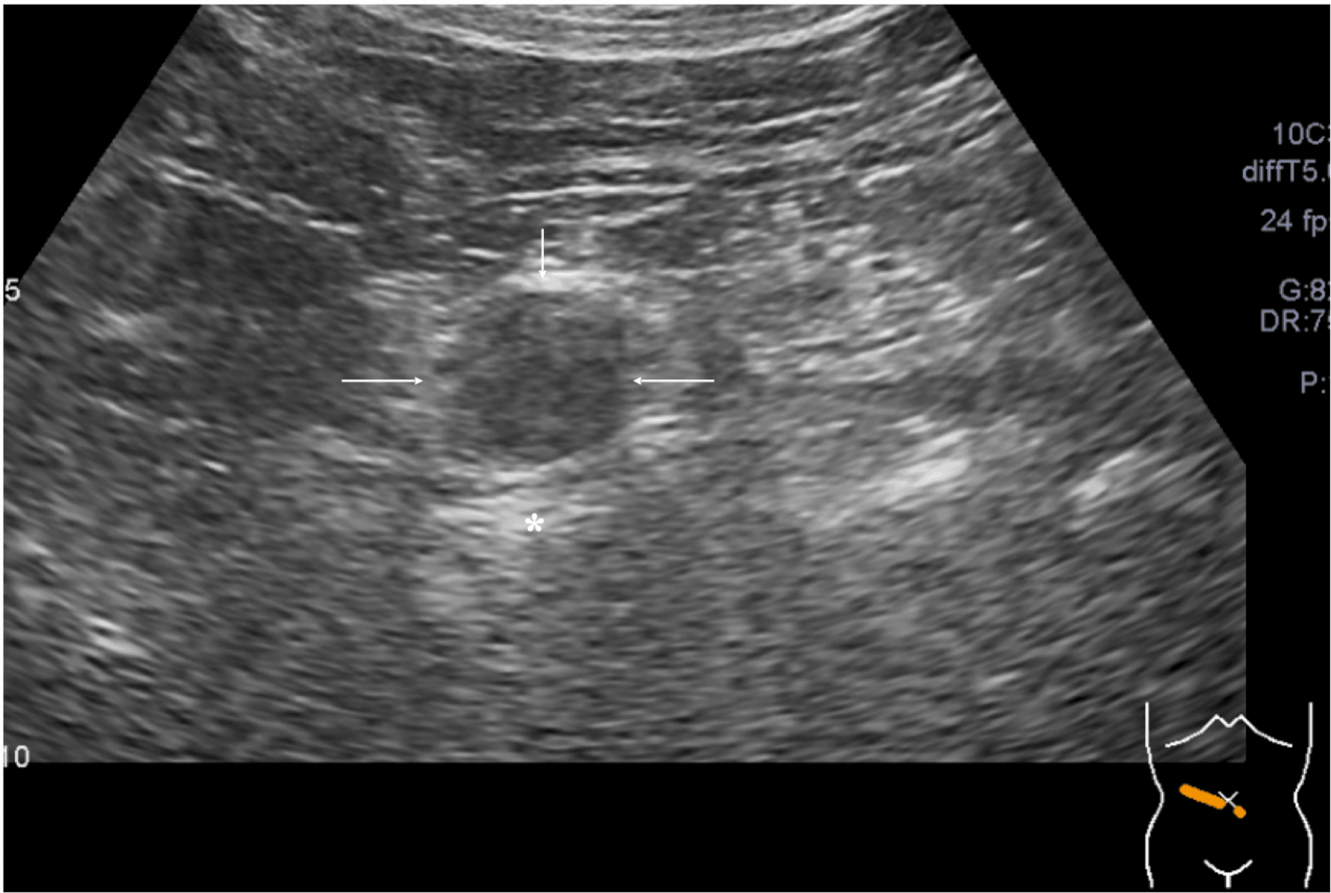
Ultrasound findings Ultrasound showed an oval mass (arrows) with internal punctate high echoes and enhanced posterior echoes (asterisk).
